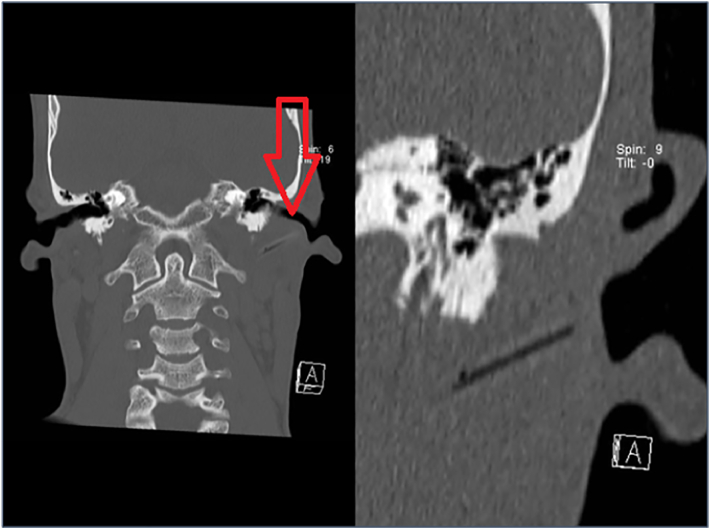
Representative coronary slices of the initial CT scan. The arrow indicates the cylindrical foreign body close to the temporal styloid process. An additional MRI was performed to identify the exact location, composition and size of the foreign body. Intraoperative Findings. After surgical exploration, a piece of wood measuring 30 × 3 mm was readily extracted.
